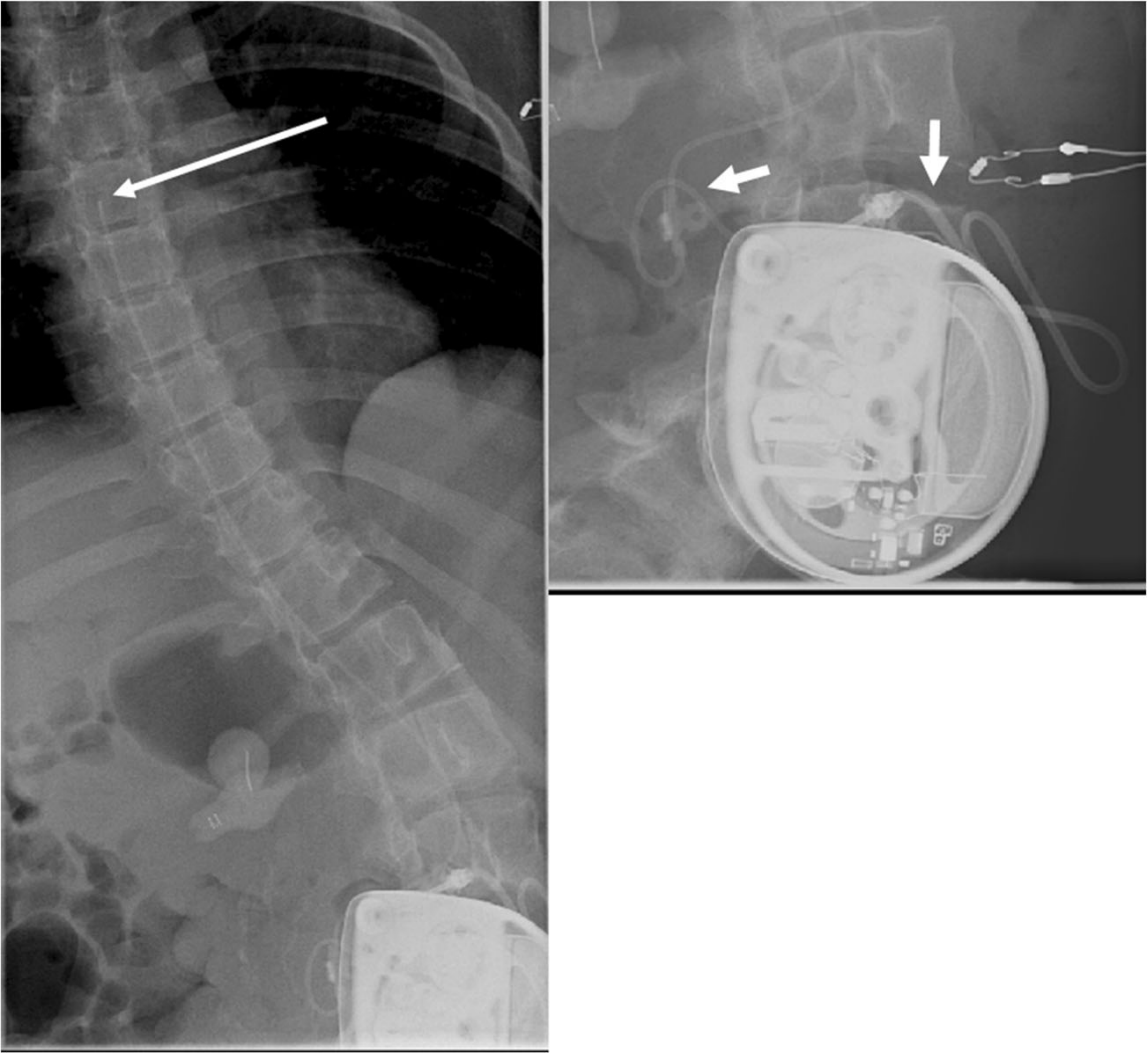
Case 4, X-ray fluoroscopy: after puncturing the access port of the pump, with correct needle position documented on the image, neither CSF can be aspirated nor contrast medium applied. The part of the tube system that is not covered by the pump is shown correctly, the tip of the catheter is projected at the level of the 6th thoracal vertebra (long white arrow). The short white arrows disclose the rolling up of the catheter
